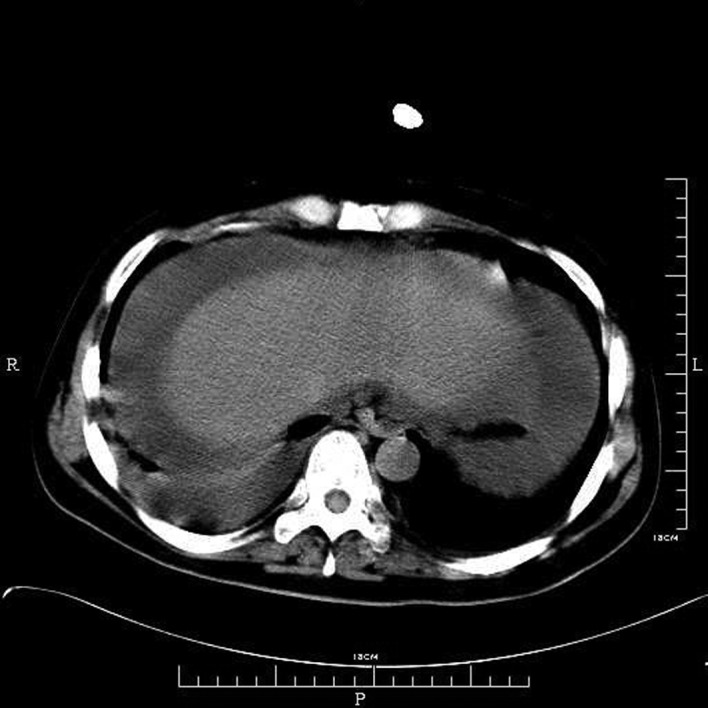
Serous effusions. CT showing the right pleural and abdominal effusions. CT, computed tomography.
